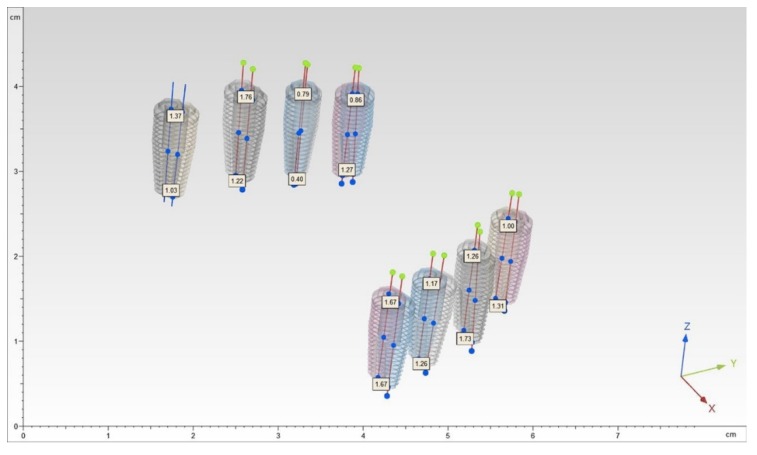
Superimposition of the preoperative and postoperative CBCTs and measurements of the deviations between the planned and the placed implants.
